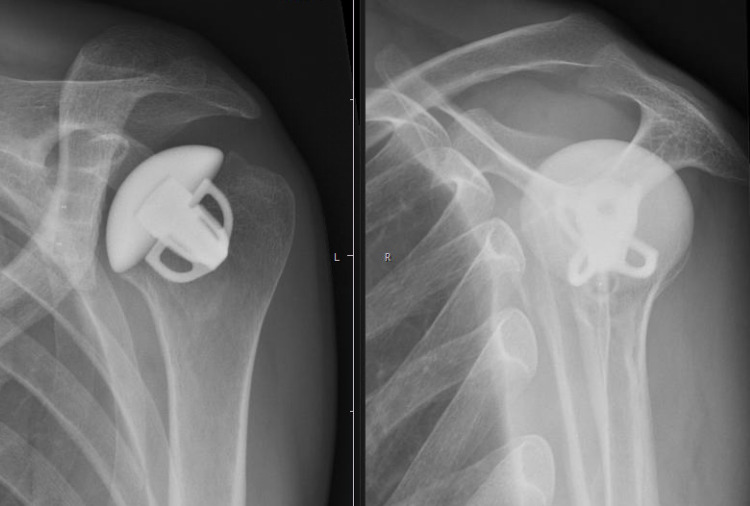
Anteroposterior radiograph (left) and Neer view (right) of the left shoulder after anatomic total shoulder replacement with a titanium short stem, a ceramic head, and a vitamin E-enhanced polyethylene glenoid component
